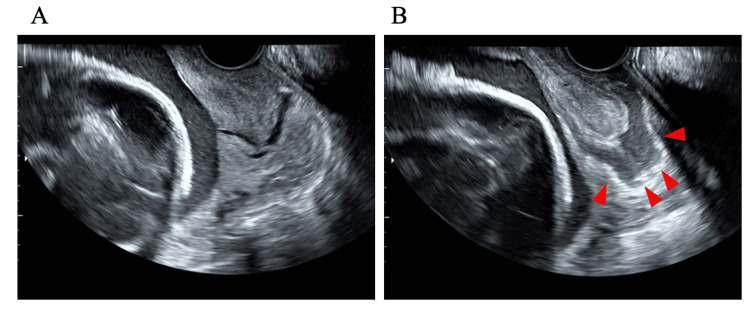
Transvaginal ultrasonography. (A) Normal uterine cervical line. (B) Hypoechoic area on the right lateral side of the cervical line.
